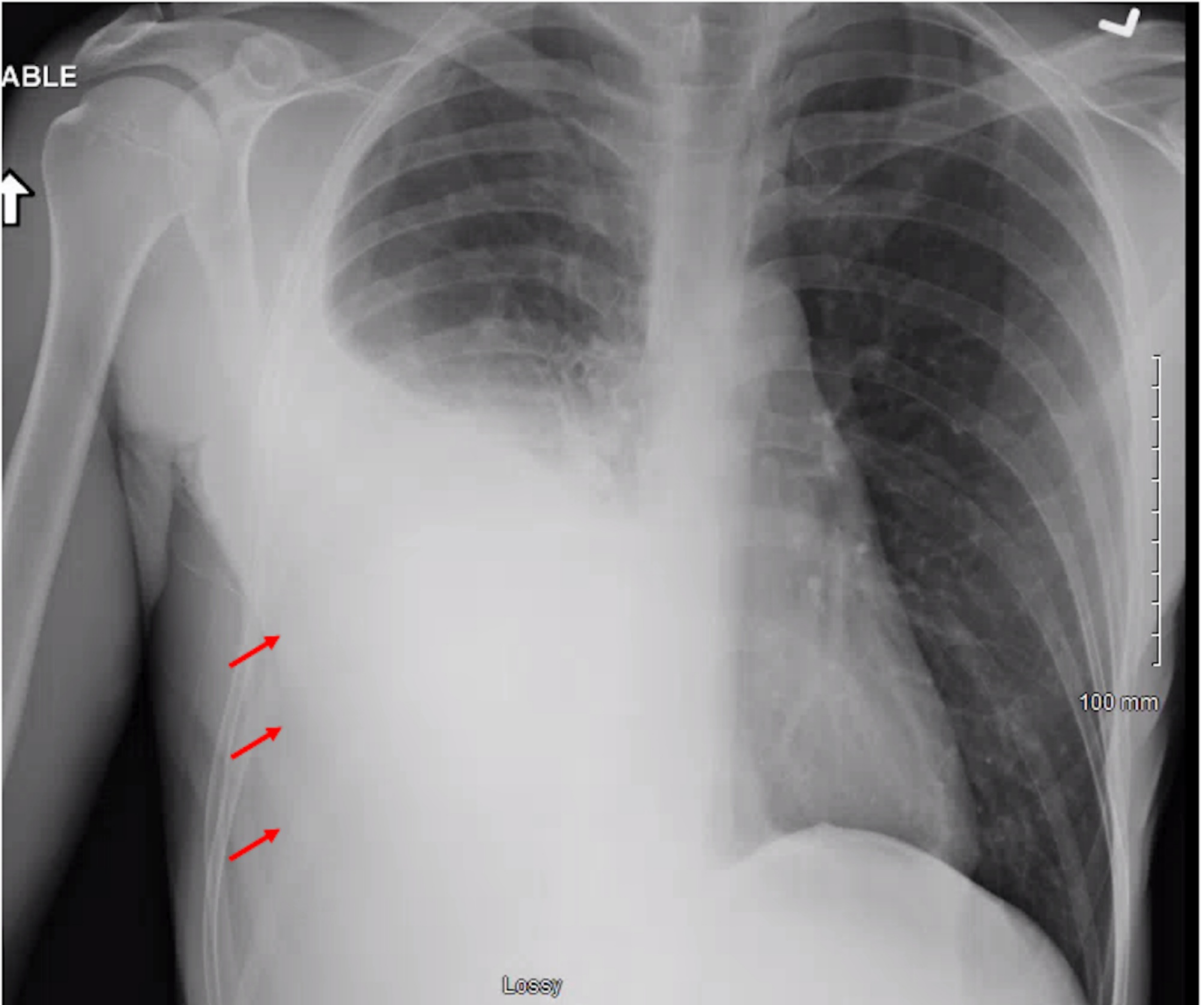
Chest X-ray revealing right-sided pleural effusion
